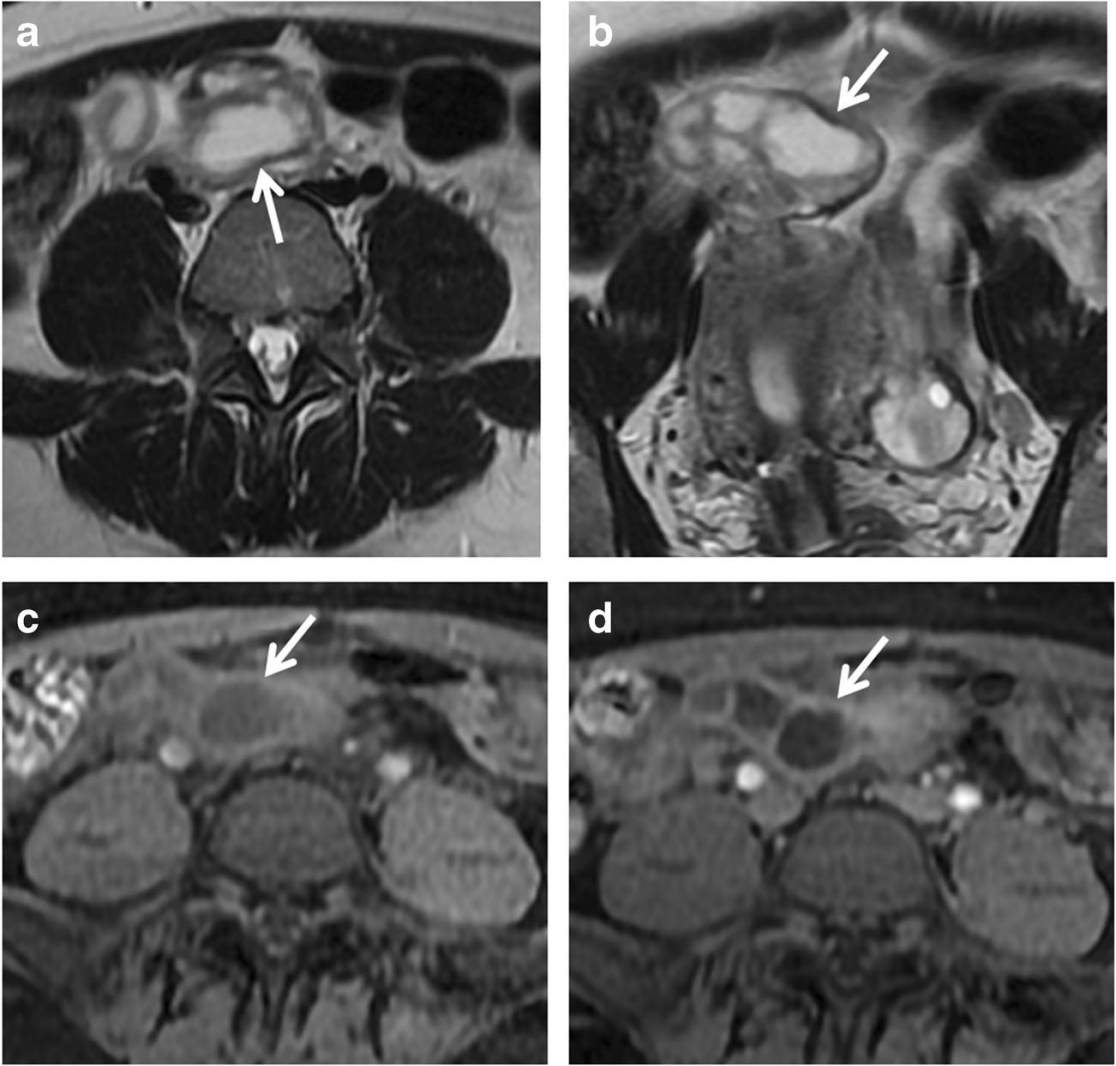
Hydrosalpinx in a 27-year-old puerperal woman. (a) Axial and (b) coronal T2-weighted MR images show a tortuous, tubular right adnexal structure with hyperintense content (*white arrows*). (c) Axial unenhanced and (d) contrast-enhanced fat-suppressed T1-weighted images show dilated tube with hypointense content and minimal parietal enhancement after contrast administration (*white arrows*)
